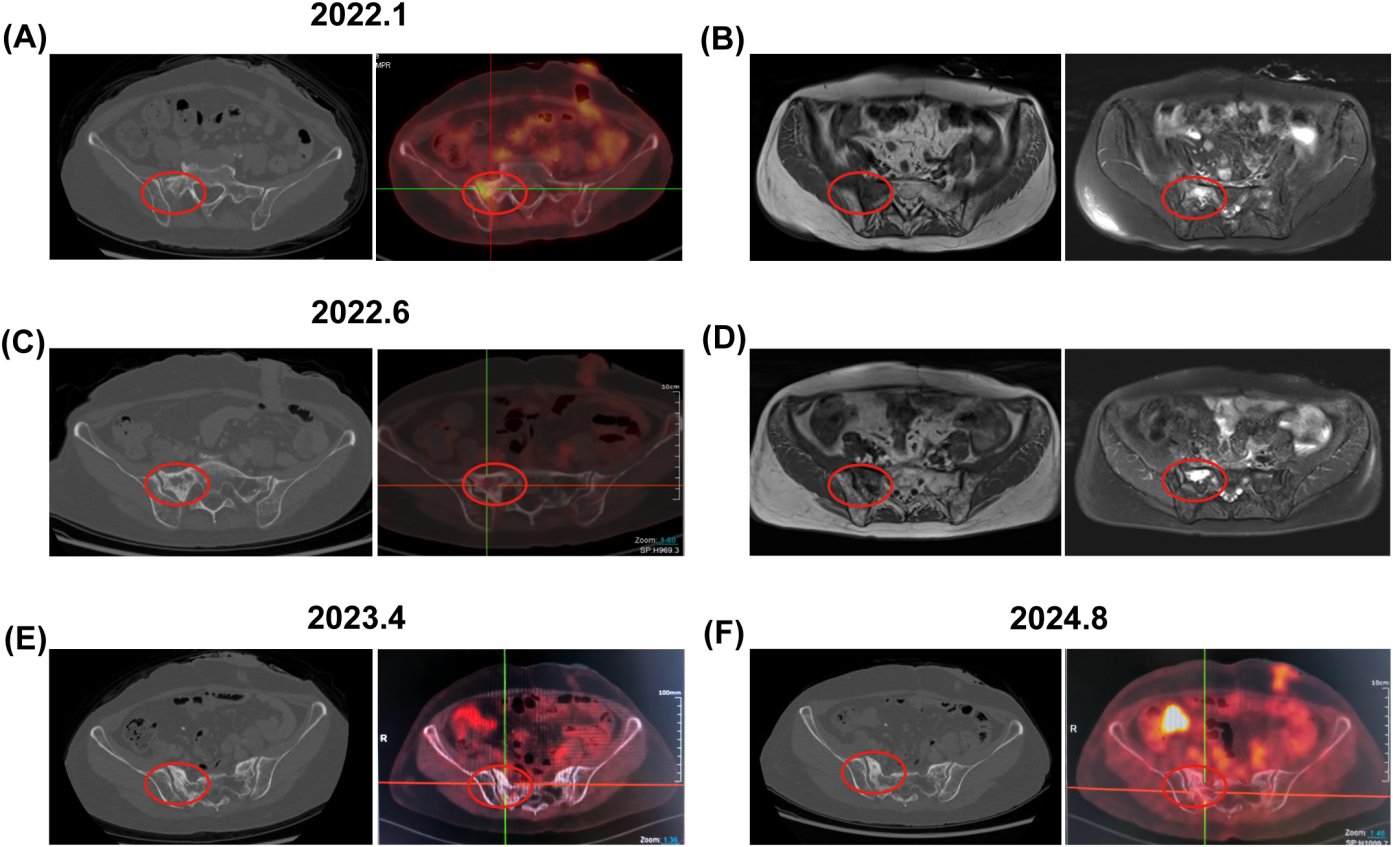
Bone changes observed in the patient from case 1. January 2022: (A) Enhanced CT and PET-CT images revealed low-density bone shadows in the sacrum, associated with increased metabolic activity (SUV 3.3). (B) An axial T1-weighted MRI image displayed low signal intensity in the right sacrum, while axial and coronal fat-suppressed T2-weighted images indicated patchy long T2 signals, suggestive of edema or infiltrative changes. June 2022: (C) Enhanced CT and PET-CT images continued to show persistent low-density bone shadows in the sacrum, albeit with decreased metabolic activity (SUV 2.8). (D) MRI findings mirrored previous observations, with low signal intensity in the right sacrum and patchy long T2 signals on axial and coronal fat-suppressed T2-weighted images. April 2023 and August 2024: (E, F) PET-CT scans revealed changes in the sacrum with no metabolic increase. The red circle highlights the area of bone change. CT, computed tomography; PET-CT, positron emission tomography-computed tomography; MRI, magnetic resonance imaging.
